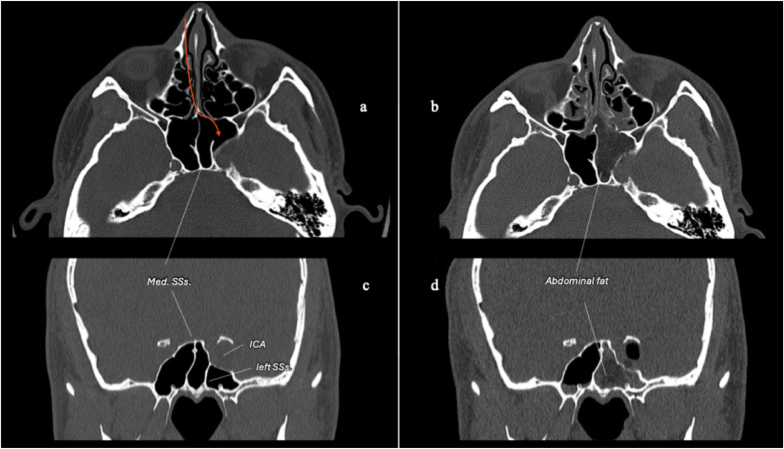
Preoperative (a, c) and postoperative (b, d) axial and coronal CT scans in the bone window demonstrating the right contralateral mononostril endonasal approach to the left cavernous sinus (CS). (a, c) The median sphenoid sinus (SS) septation lies in the midline, while a left SS septation inserts onto the left cavernous internal carotid artery (ICA) prominence. These septations represent important intrasphenoidal landmarks. (b, d) Through a left transrostral corridor, only a left hemi-sphenoidotomy is performed. The parasellar mini-craniotomy is reconstructed, the left hemisinus is filled with abdominal fat, and the rostral mucosa is sutured. Nasal anatomy is preserved as much as possible. *Abbreviations:* Med. SSs., median sphenoid sinus septation; SSs., sphenoid sinus septation.
